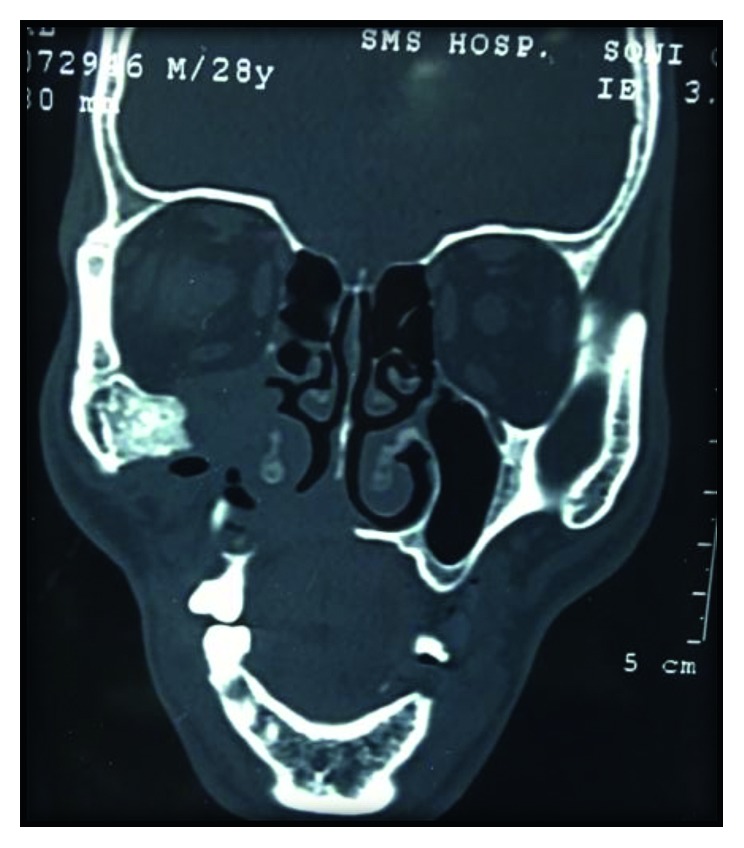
Axial CT view showing maxillectomy defect.
